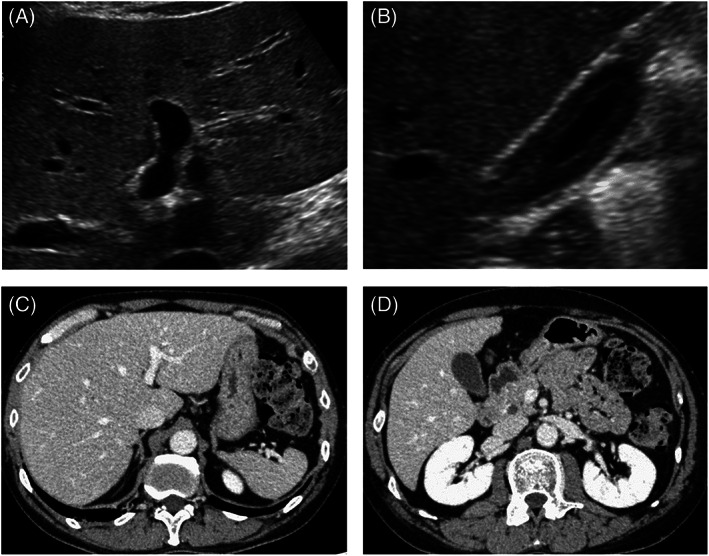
Radiological findings of Case 2. (A) Ultrasonography reveals no apparent abnormality in the liver. (B) Diffuse thickening of the gallbladder wall is present. (C and D) Contrast‐enhanced CT reveals no abnormal findings
